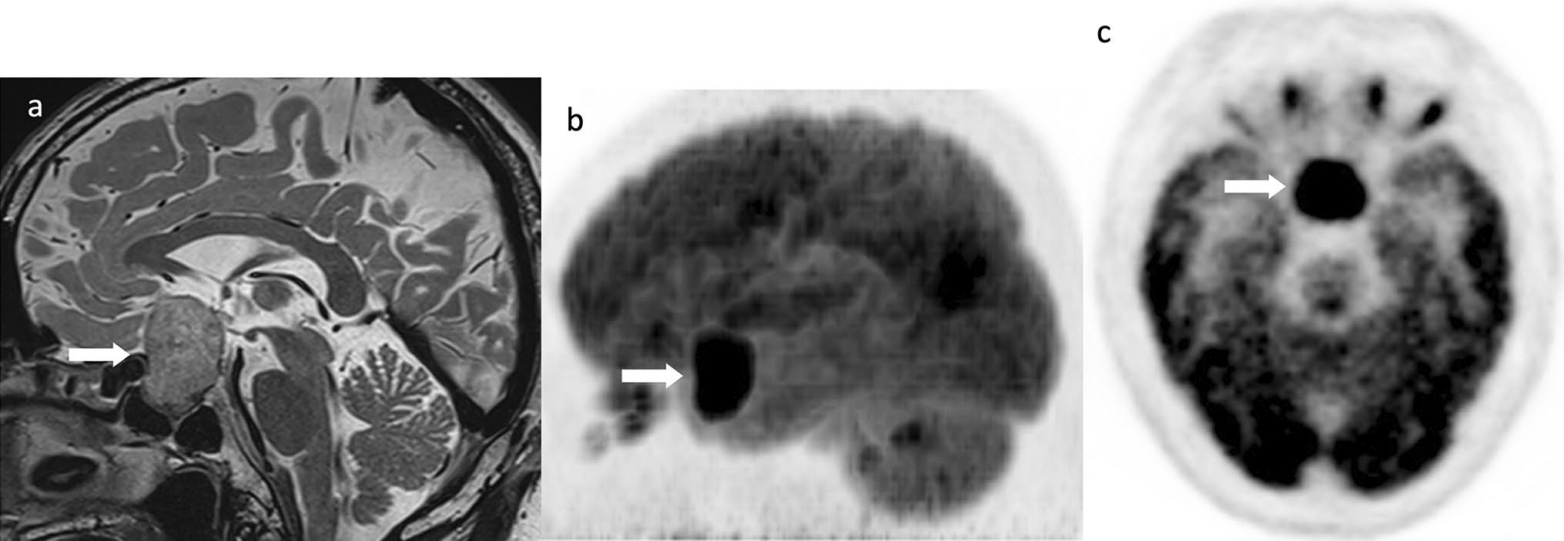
Fluorodeoxyglucose (FDG)-positron emission tomography accumulation in a PitNET/pituitary adenoma. A male patient in his 50 s, with a nonfunctioning PitNET/pituitary adenoma. a Sagittal T2WI revealed a mass within the sella turcica to the suprasellar region (arrow). b, c Marked FDG accumulation in the mass (arrow) (SUVmax = 20.1). Courtesy of Dr. Yuji Nakamoto
